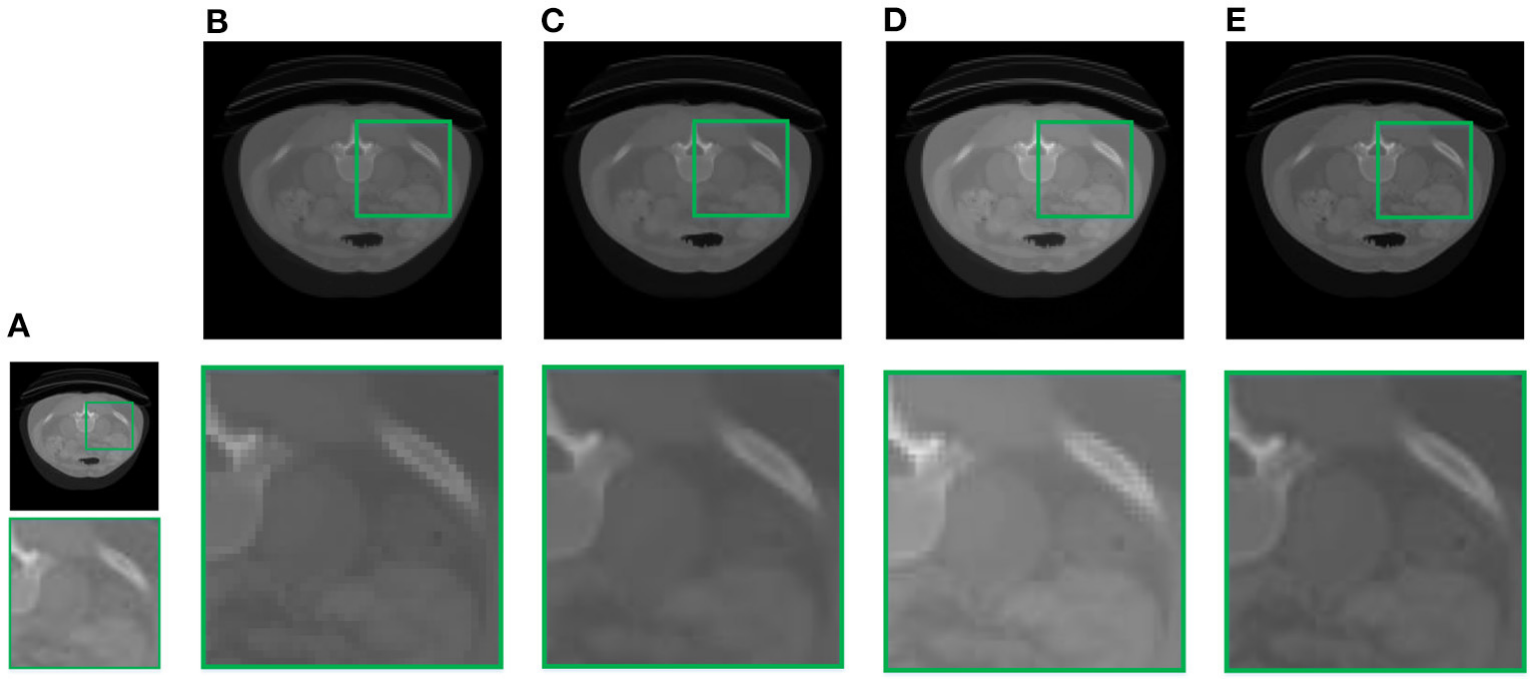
Reconstruction results and corresponding details of contrast-enhanced abdominal CT data from NIH pancreas segmentation database: (A) LR band with downsampling factor 2, (B) NN, (C) LRTV, (D) TF-SISR, (E) NNTDTV.
